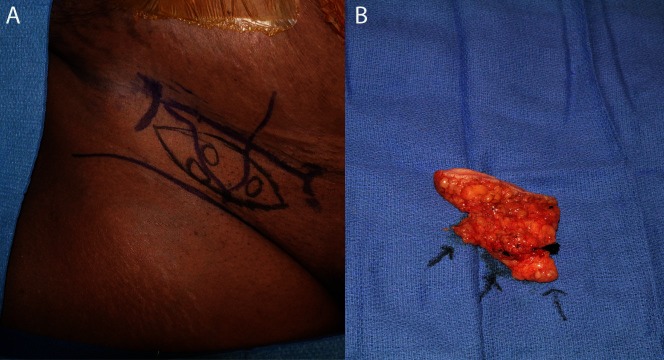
Skin markings for the groin-based lymphatic flap with nodes identified by lymphoscintigraphy and ICG (A). The dissected flap is shown with arrows indicating lymph nodes (B).
